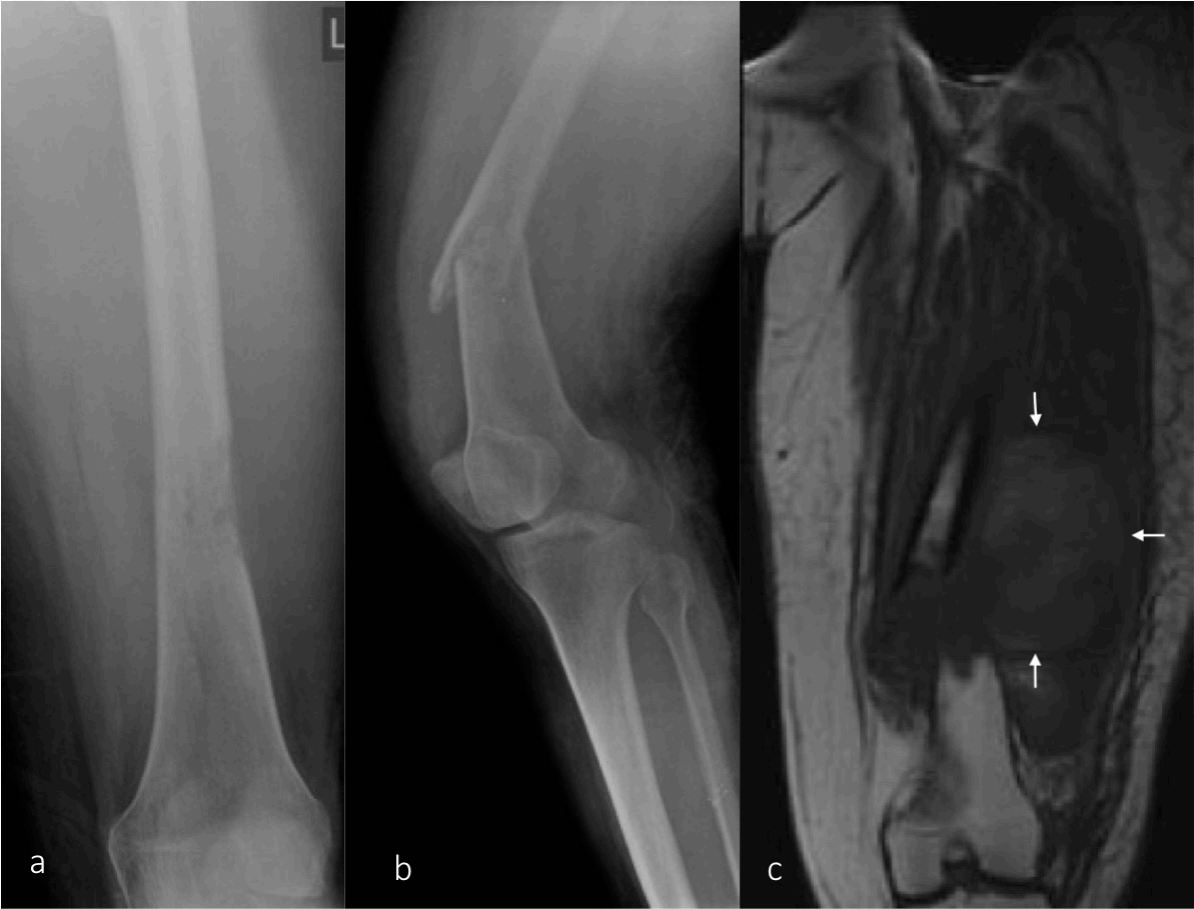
Pathological fracture/ (a) AP radiograph of the femur demonstrating a lucent lesion in the distal femur with permeative pattern of bone destruction suggesting an aggressive lesion. (b) Lateral view of the knee joint including the distal femur demonstrating a pathological fracture through the lesion. (c) T1 coronal MRI image showing aggressive lesion replacing the normal marrow fat at the fracture site with a large adjacent soft tissue component. AP, anteroposterior.
